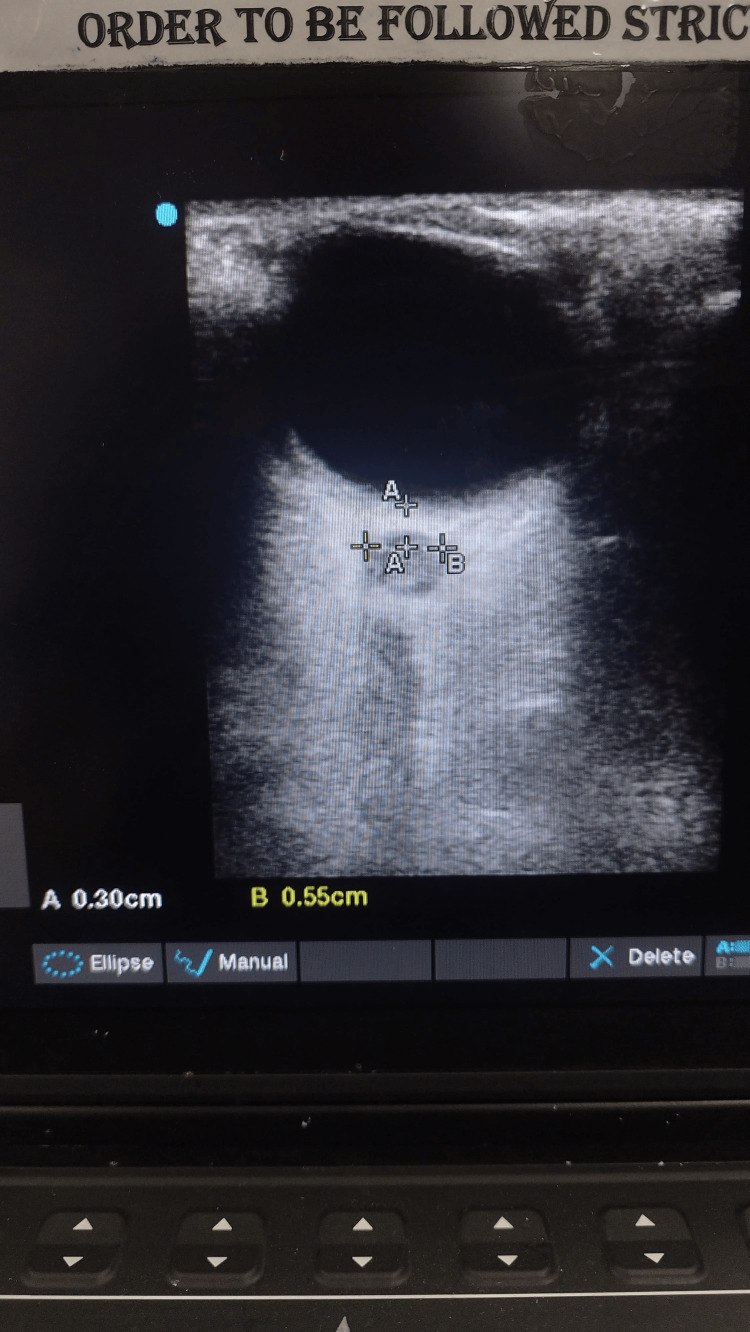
Optic Nerve Sheath Diameter (ONSD) Ultrasound The optic nerve sheath diameter (ONSD) is measured 3mm behind the retina, from which point a transverse line is drawn from the inner edge to the inner edge of both vertical hypoechoic lines.
